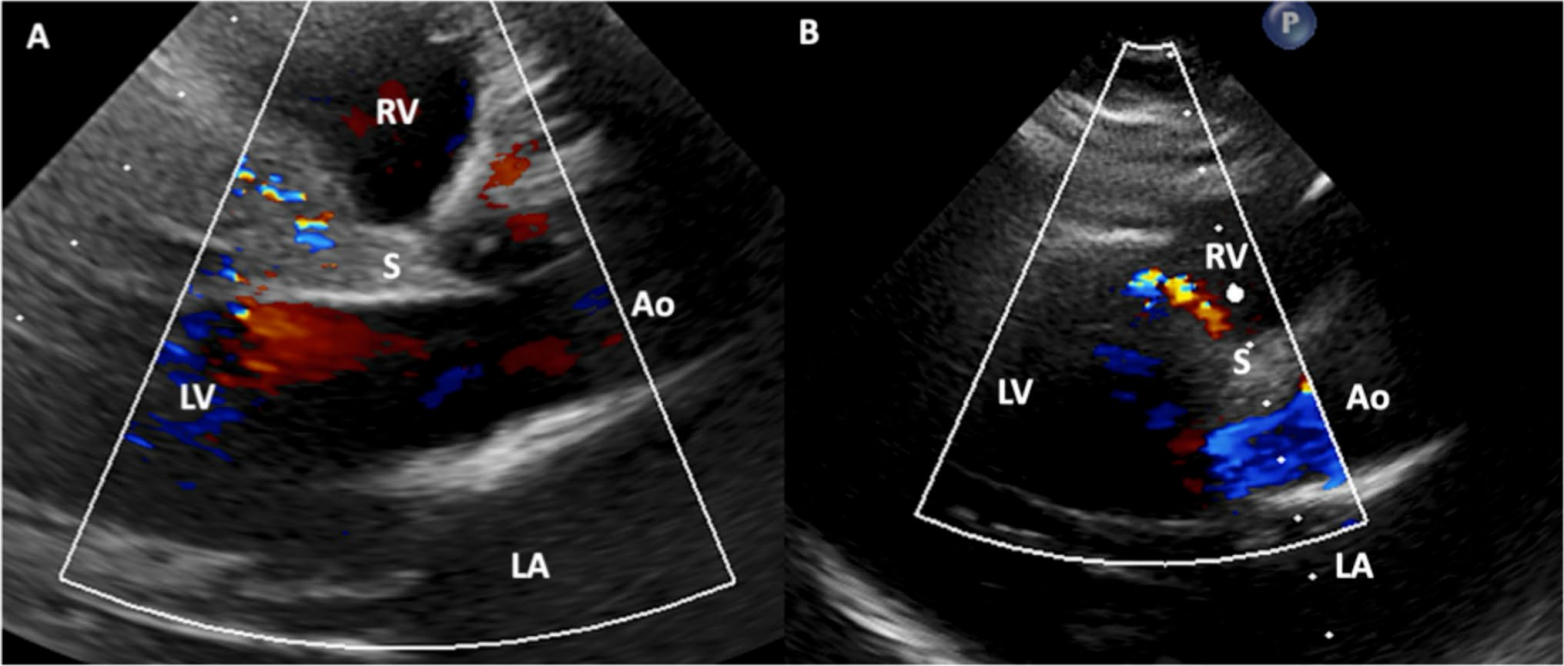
Figure 1 shows the abnormal septal TTE color doppler signal due to an excessive septal collateral system. *Ao* aorta, *LA* left atrium, *LV* left ventricle, *S* interventricular septum, *RA* right atrium, *RV* right ventricle, *TTE* transthoracic echocardiography
